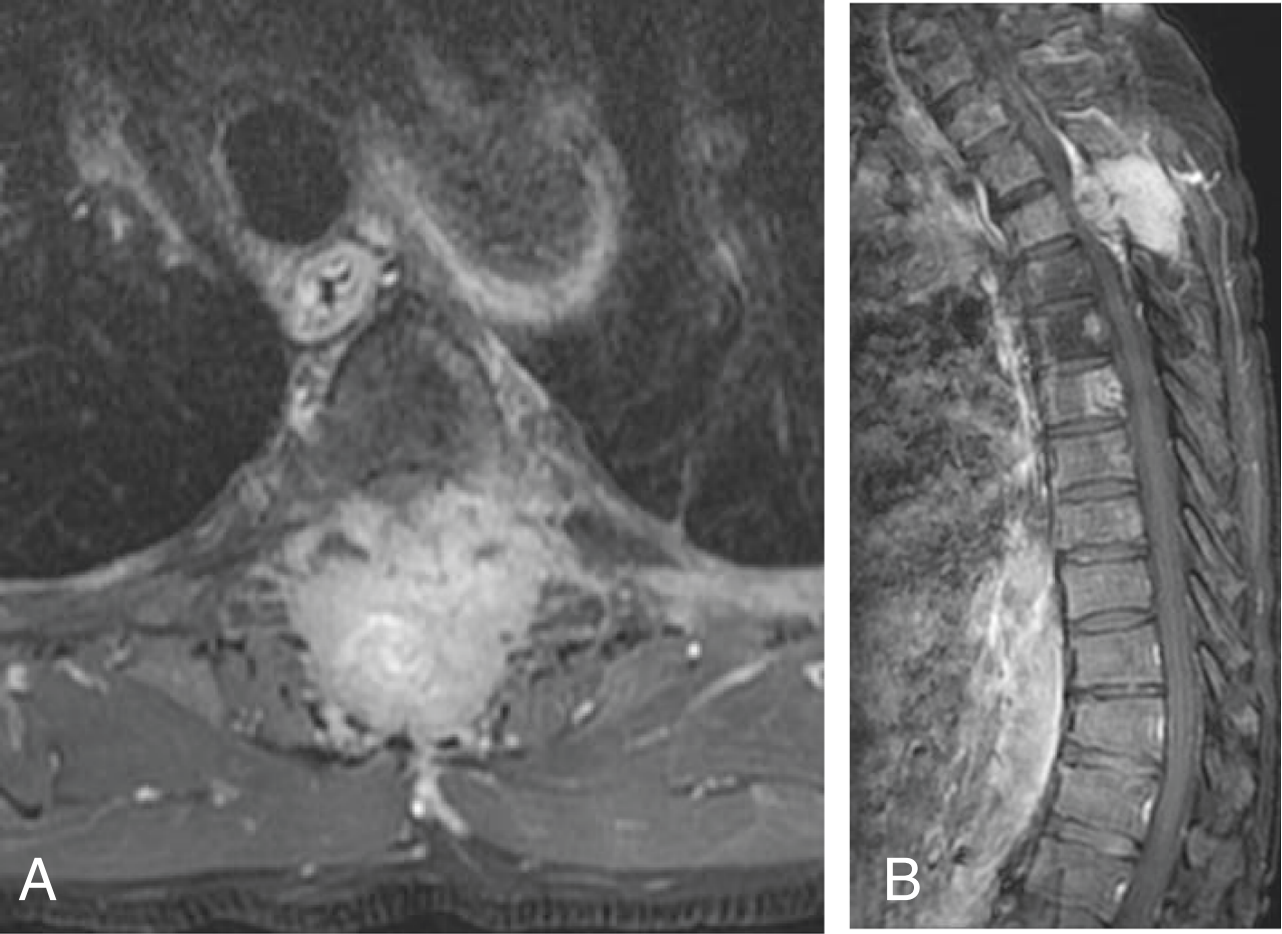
A 69-year-old male lung cancer patient with T3, T6 metastases underwent spinal surgery; his preoperative palsy score was 0 (Frankel B). Axial (a) and sagittal (b) T1-weighted MR images with contrast enhancement demonstrate severe cord compression at T3. His postoperative palsy score was 1 (Frankel C), and he survived 289 days after spinal surgery
